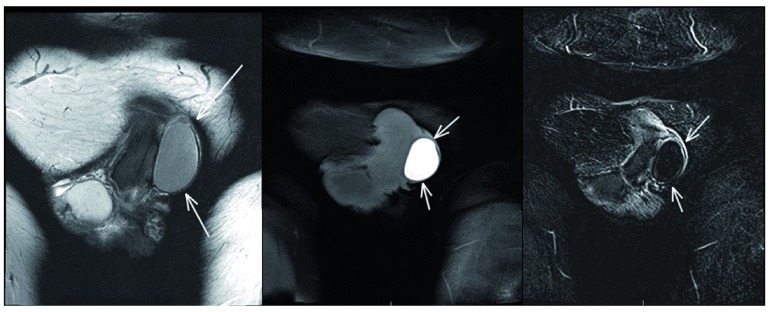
a: T-2 MRI reveals non-enhancing 4.1 cm lesion abutting the left corpus cavernosa and exerting mild compression on the left corpus cavernosum.
